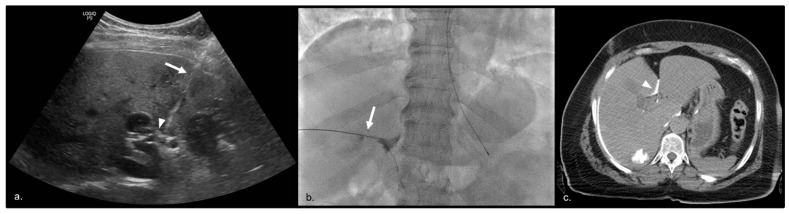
Using ultrasound guidance, a 22-gauge needle (arrow) was placed at the bifurcation of the main portal vein (arrowhead) (a). Contrast was injected into the 22-gauge needle (arrow) under fluoroscopy to confirm extravascular location (b). CT imaging was then obtained, which showed contrast surrounding the portal vein (arrowhead) (c).
